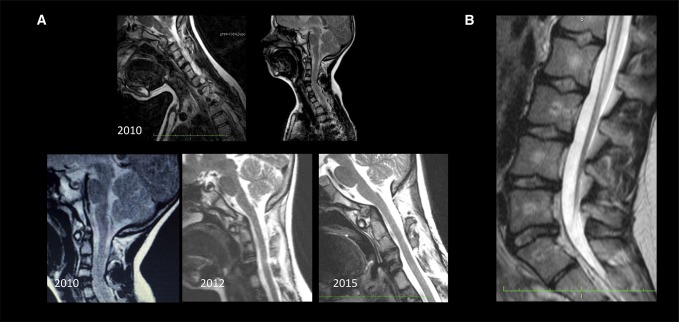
MRI of the cervical spine demonstrating the instability at C4/5 (A, upper panel) and the progressive horizontal instability at C1/2 over a period of 5 years (A, lower panel). The conus medullaris is located at the level of L1 (B).
